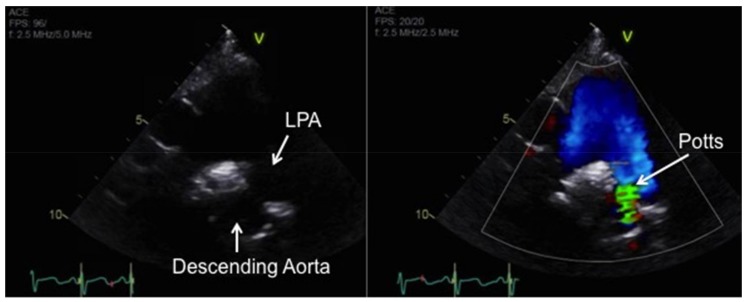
Echocardiogram of the Potts shunt in a patient with severe IPAH by 2D imaging (left) and with color Doppler (right). LPA, left pulmonary artery.
